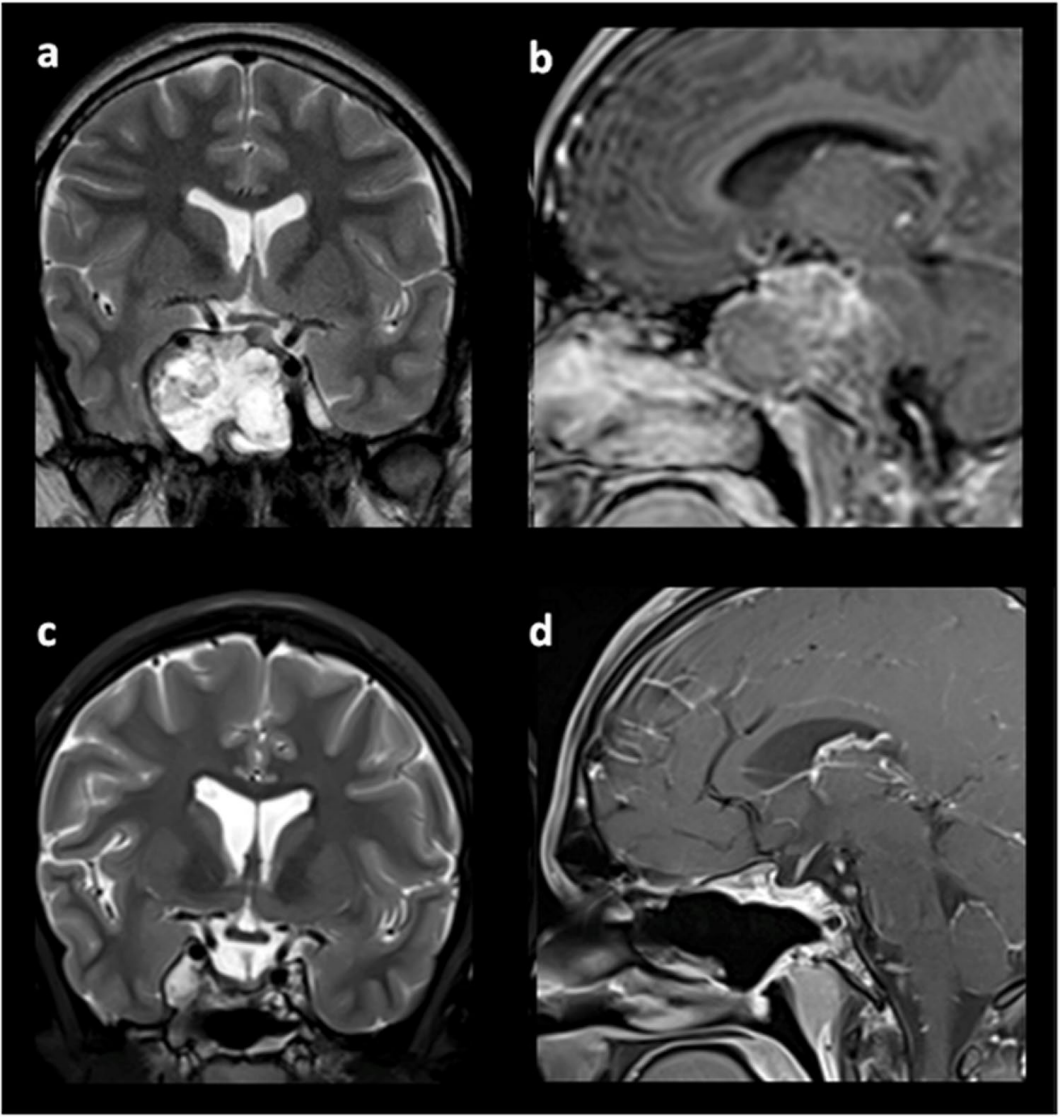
Sphenopetroclival chondrosarcoma in a 16-year-old male: a, b coronal T2-weighted and sagittal T1-weighted contrast-enhanced MRI preoperative imaging; c, d coronal and sagittal postoperative imaging, showing gross total resection
